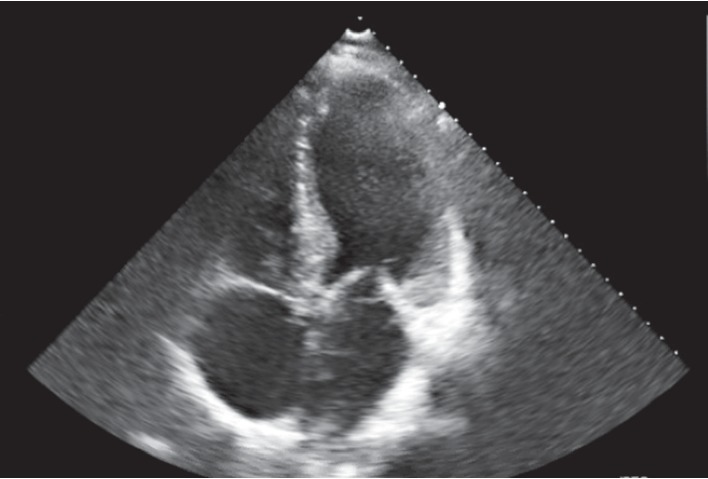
Echocardiogram showing normal biventricular dimensions with biatrial dilation with no significant valvular abnormalities.
